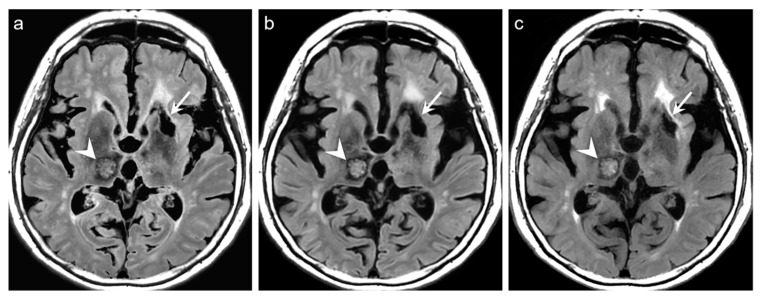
Representative case of lesion conspicuity on DL-FLAIR image. Intracranial hemorrhage (ICH) (arrowheads) is well visualized in the right thalamus on the native synthetic FLAIR (a), DL-FLAIR (b), and conventional FLAIR images (c). In particular, the thalamic ICH is more conspicuously delineated on DL-FLAIR (b) than on the native synthetic FLAIR image (a). However, the conspicuity of the true hyperintensity around the encephalomalacia in the left basal ganglia (arrows on a, c) is slightly decreased on DL-FLAIR image (arrow on b).
